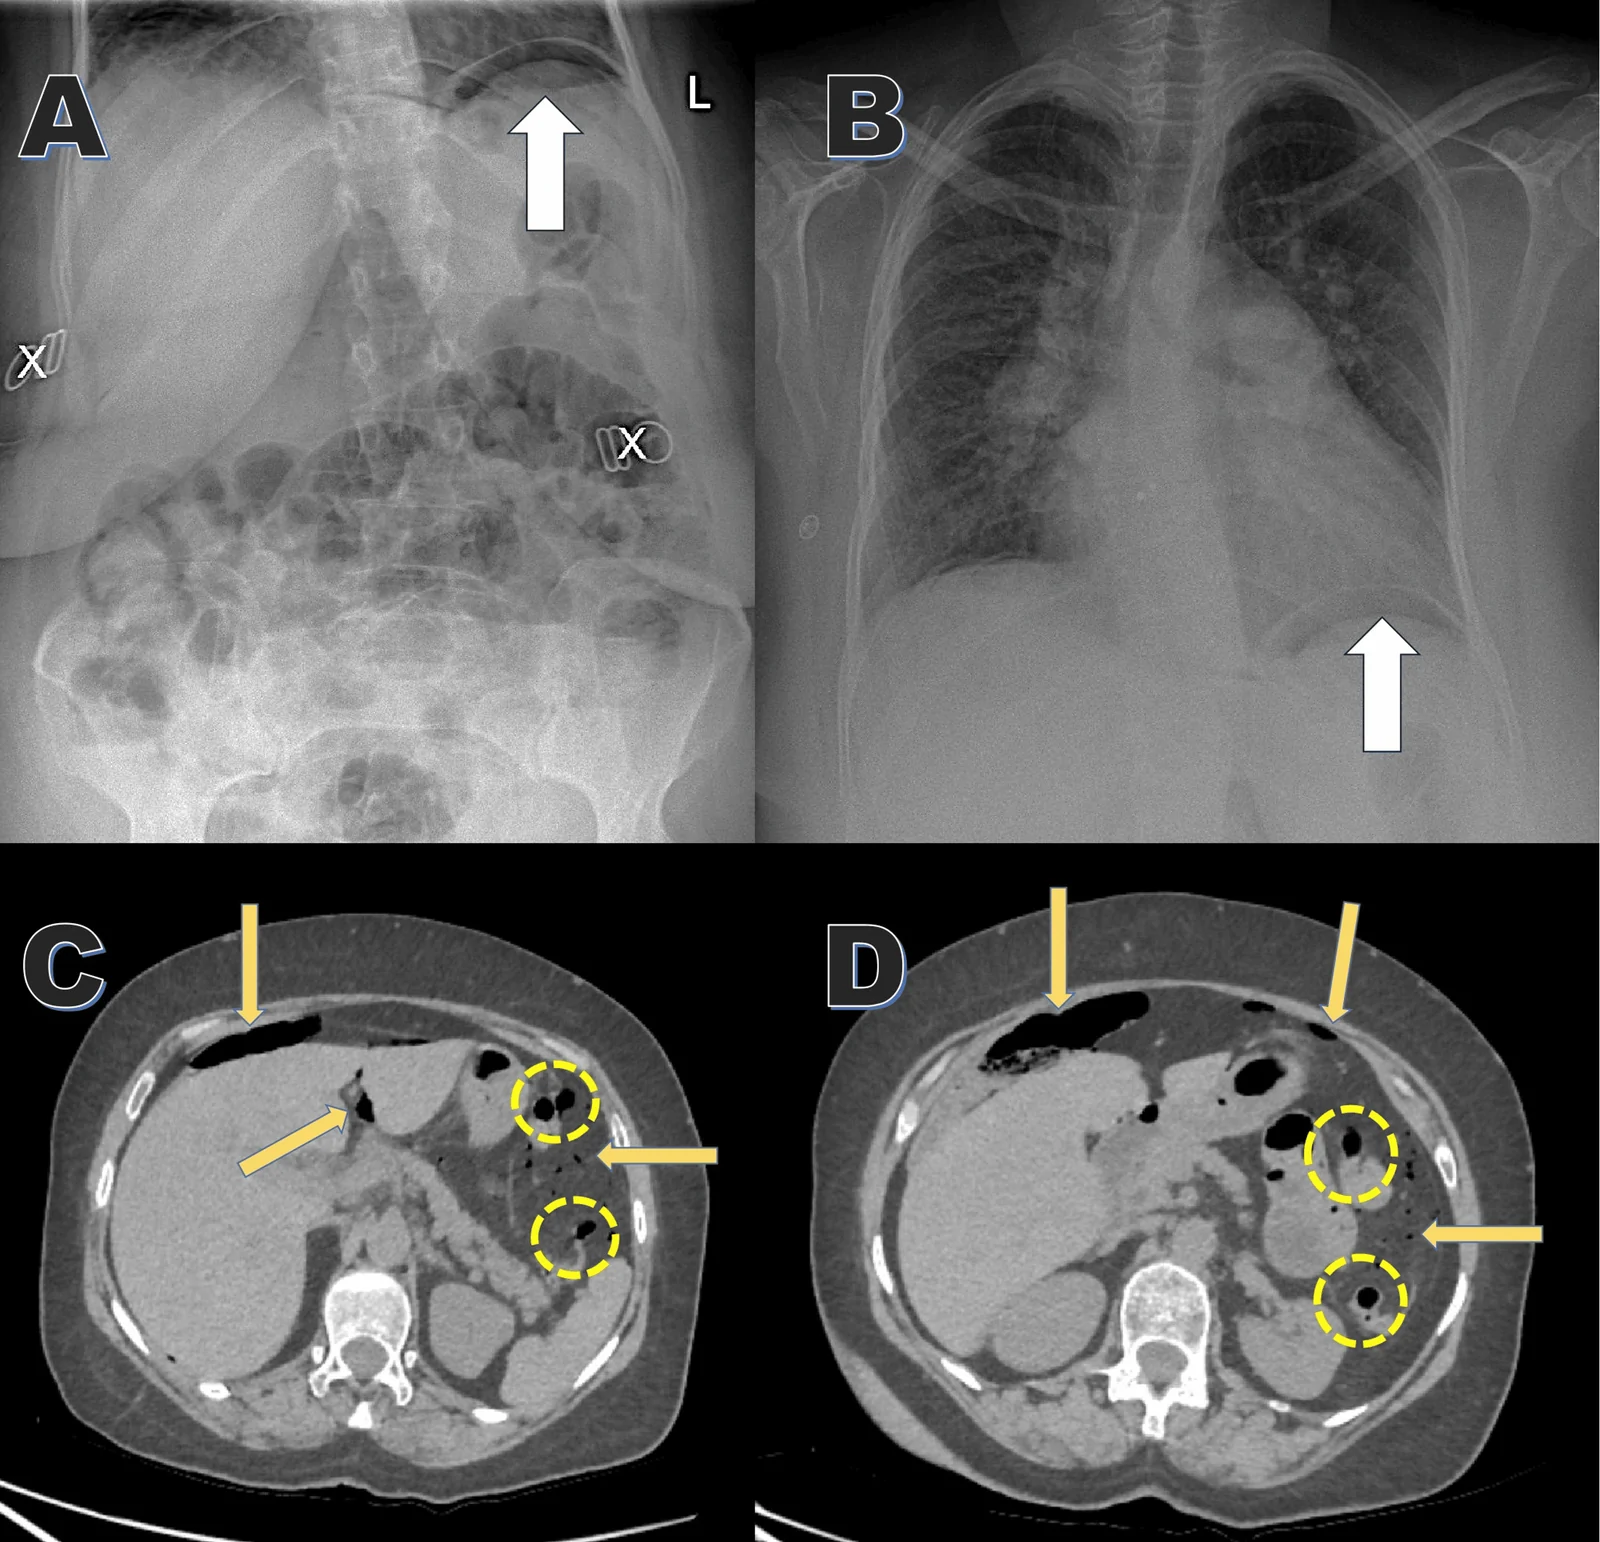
X-ray and CT images (A) X-ray of the abdomen, anteroposterior (AP) erect view; (B) X-ray of the chest, posteroanterior (PA) view, showing air under the left copula of the diaphragm (white arrows); (C-D) Plain computed tomography (CT) study of the abdomen, axial cuts, showing evidence of pneumoperitoneum (yellow arrows) and multiple jejunal diverticulae (yellow circles).
